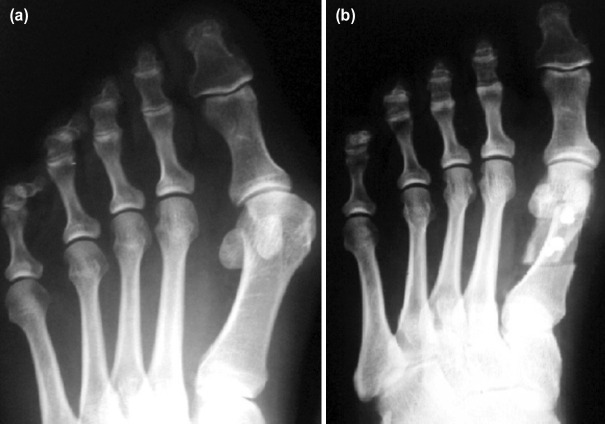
(a) The pre-surgery radiograph. (b) The post-surgery radiograph after performing a SCARF osteotomy.
